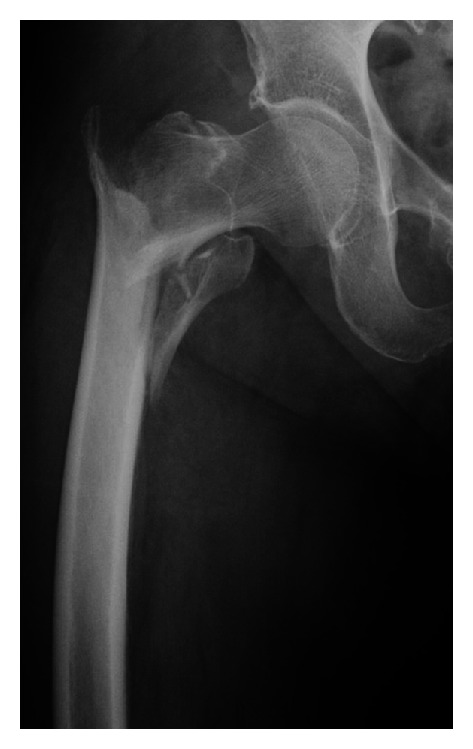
Radiograph showing an unstable trochanteric fracture of the right femur classified as 31-A2.2 according to the Orthopaedic Trauma Association classification.
